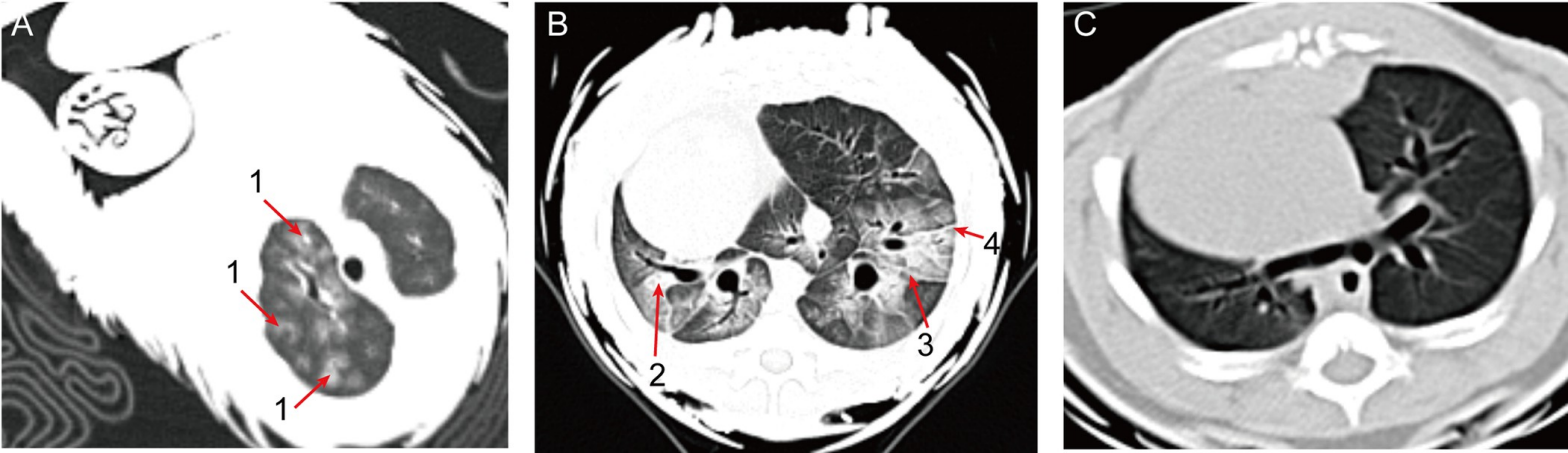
Chest CTs of two PCoV-GD-positive and one virus negative pangolin. (A) Multiple ground-glass opacities in bilateral lungs in one pangolin that was positive to both PCoV-GD and Sendai virus. Arrowheads 1: ground-glass opacities. (B) Bilateral focal consolidation, lobar consolidation, and patchy consolidation were clearly observed in the second PCoV-GD positive pangolin, which was negative for other viruses. Arrowheads 2: air bronchogram; Arrowheads 3: fibrotic streaks; Arrowheads 4: subpleural transparent line. (C) Chest CT of a virus-negative pangolin. This pangolin was negative to PCoV-GD, Sendai virus, pestivirus and parvovirus.
